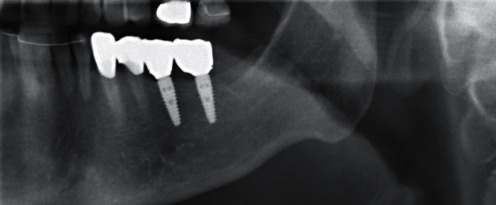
X-ray check at the crown fitting.
